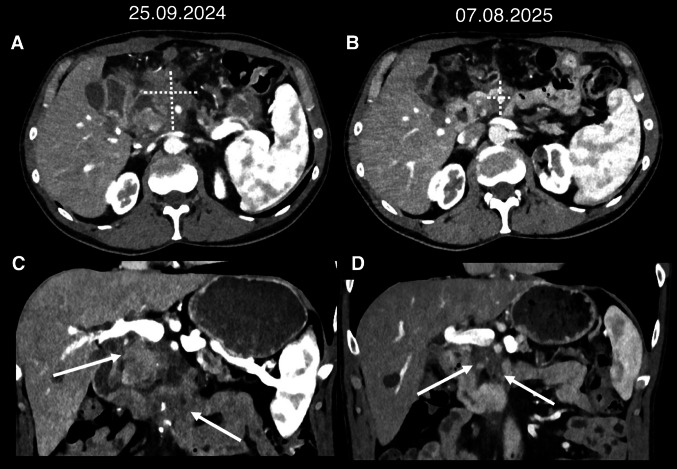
Arterial phase images of photon-counting CT scans before the initiation of pembrolizumab (A, C) and after 11 months of treatment (B, D). (Top) Axial plane reconstructions with measurements: A 5.5 × 6.4 cm, B 2.1 × 2.2 cm. (Bottom) coronal plane reconstructions: arrows point to the tumor in the pancreatic head, which can be distinguished from the pancreatic parenchyma due to its hypodense appearance
